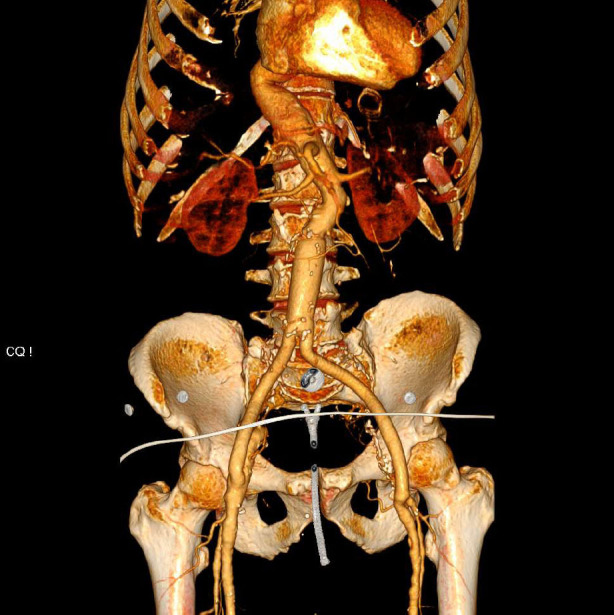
Postoperative computed tomography angiography with contrast showing complete sealing of the aneurysm and no endoleak detected.
